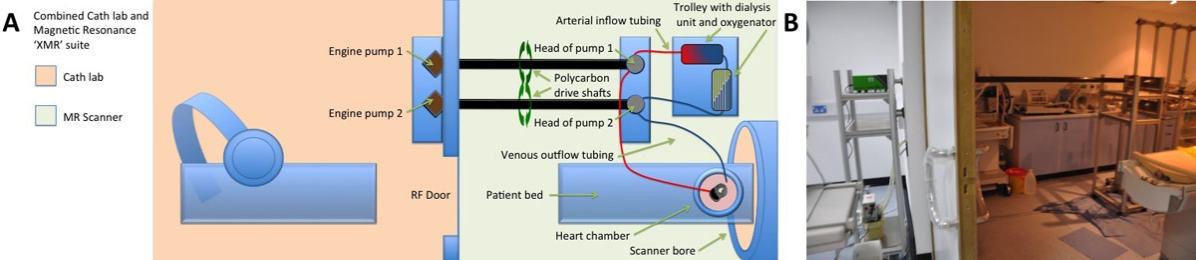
The novel set-up allows the operation of the system through wave guides from the X-Ray room (Cath lab) of the XMR suite. All electrical and magnetic parts remain in the X-Ray room. The pump engines are connected to the custom-made MR compatible pump heads using polycarbon drive shafts through custom made connectors that are fitted in the wave guides.
